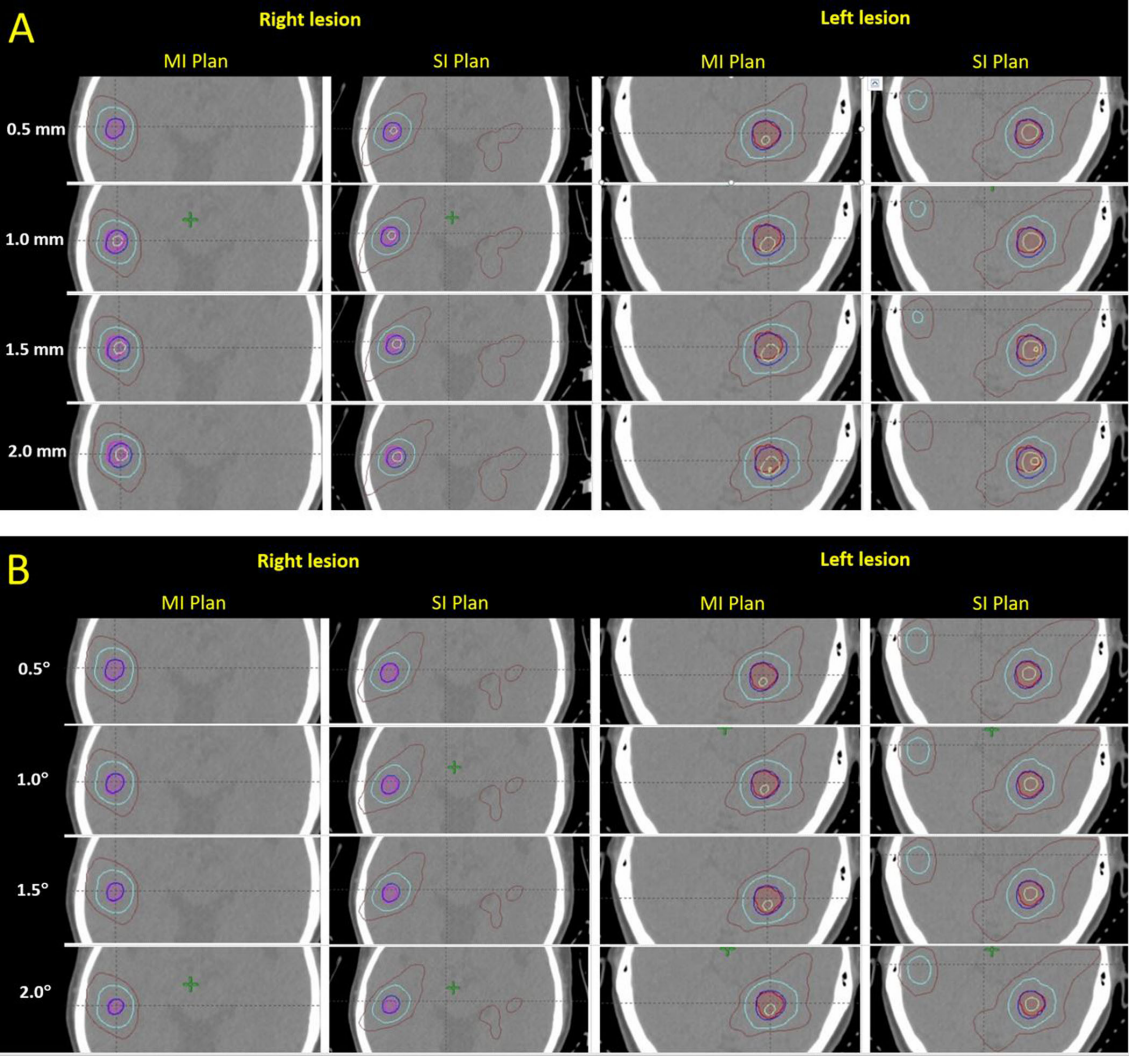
Comparison of the target (right and left brain lesions) dose distributions in axial computed tomography planes under different magnitudes of (a) translational isocenter shifts and (b) rotational isocenter shifts between MI and SI plans. Red line/pink line = PTV, blue line = prescribed isodose level (80%)
